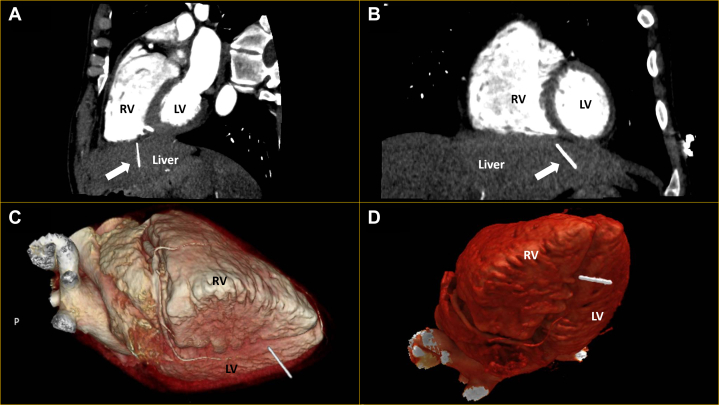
Cardiac-Gated Computed Tomography With 3D Reconstructions Cardiac-gated computed tomography in oblique sagittal (A) and coronal (B) views, as well as 3D reconstructions (C and D) demonstrate the 25-mm needle (white arrow) in the left liver lobe oriented toward the heart and 60-mm deep from the skin surface. LV = left ventricle; RV = right ventricle.
